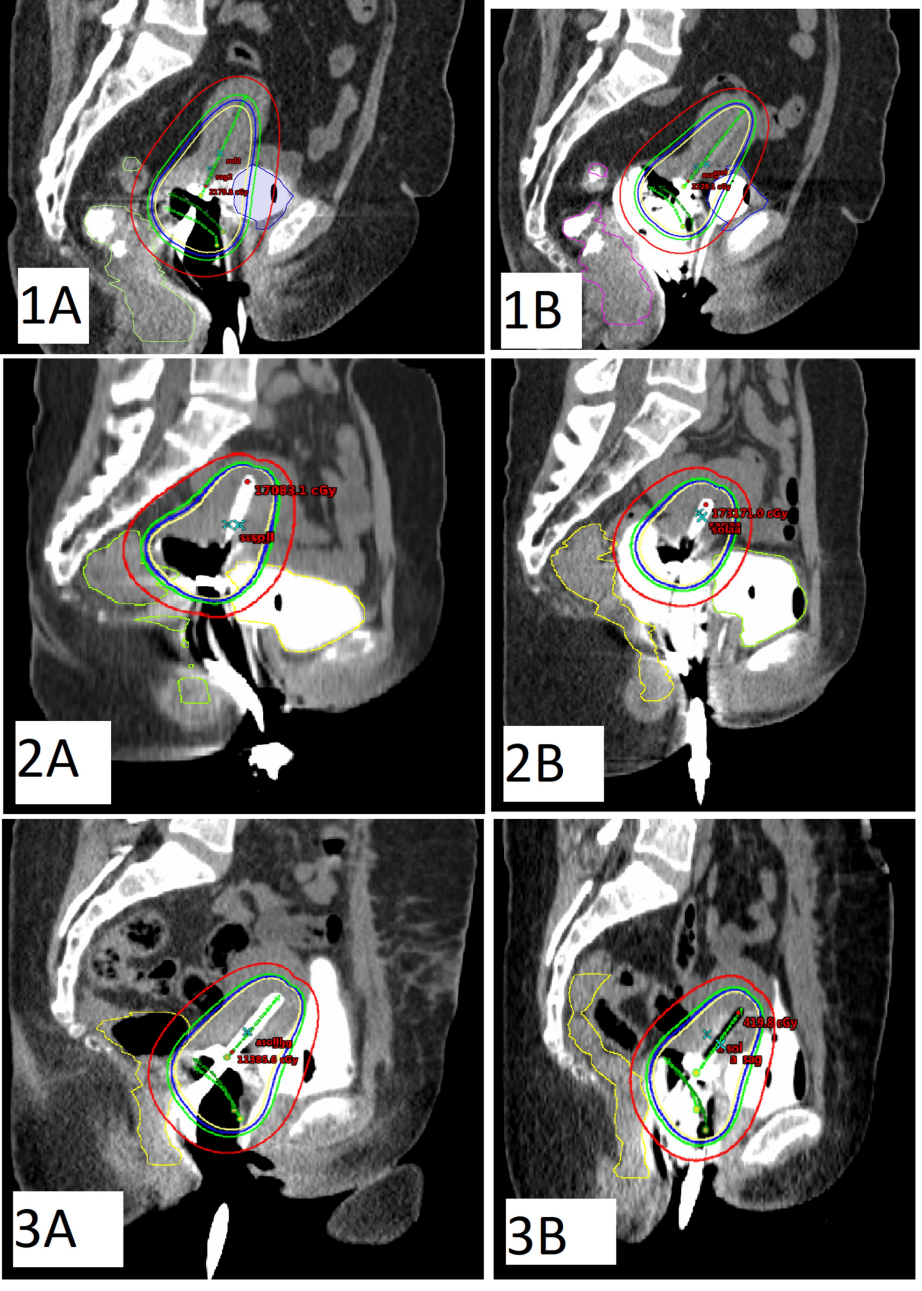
Sagittal CT images of the SP and VGP plan for three patients The images labeled with “A” next to the patient numbers correspond to the SP, while those labeled with “B” correspond to the VGP plan. Dose distributions: the yellow line represents 100% isodose; the blue line represents 90% isodose; the green line represents 80% isodose; and the red line represents 50% isodose. SP: Standard plan; VGP: Vaginal gauze packing
